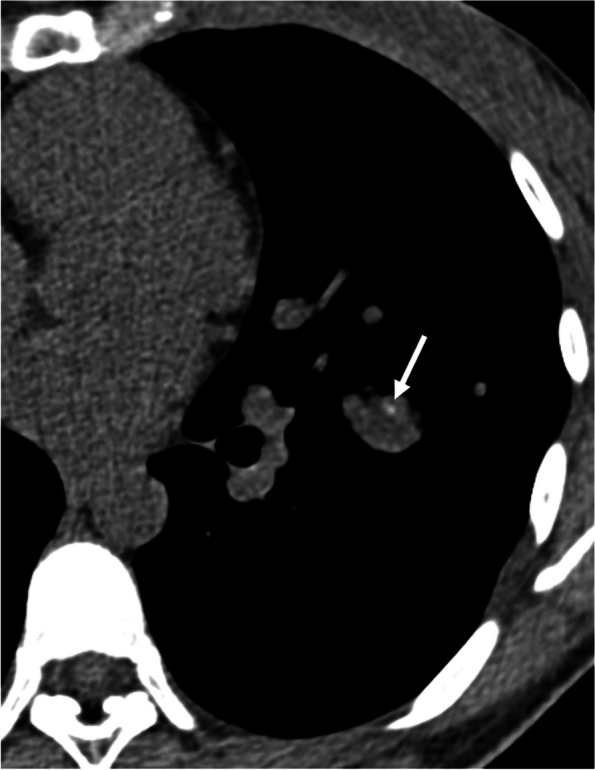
Pulmonary sclerosing pneumocytoma in a 25-year-old woman. CT image shows punctate calcifications within the large nodule (arrow)
